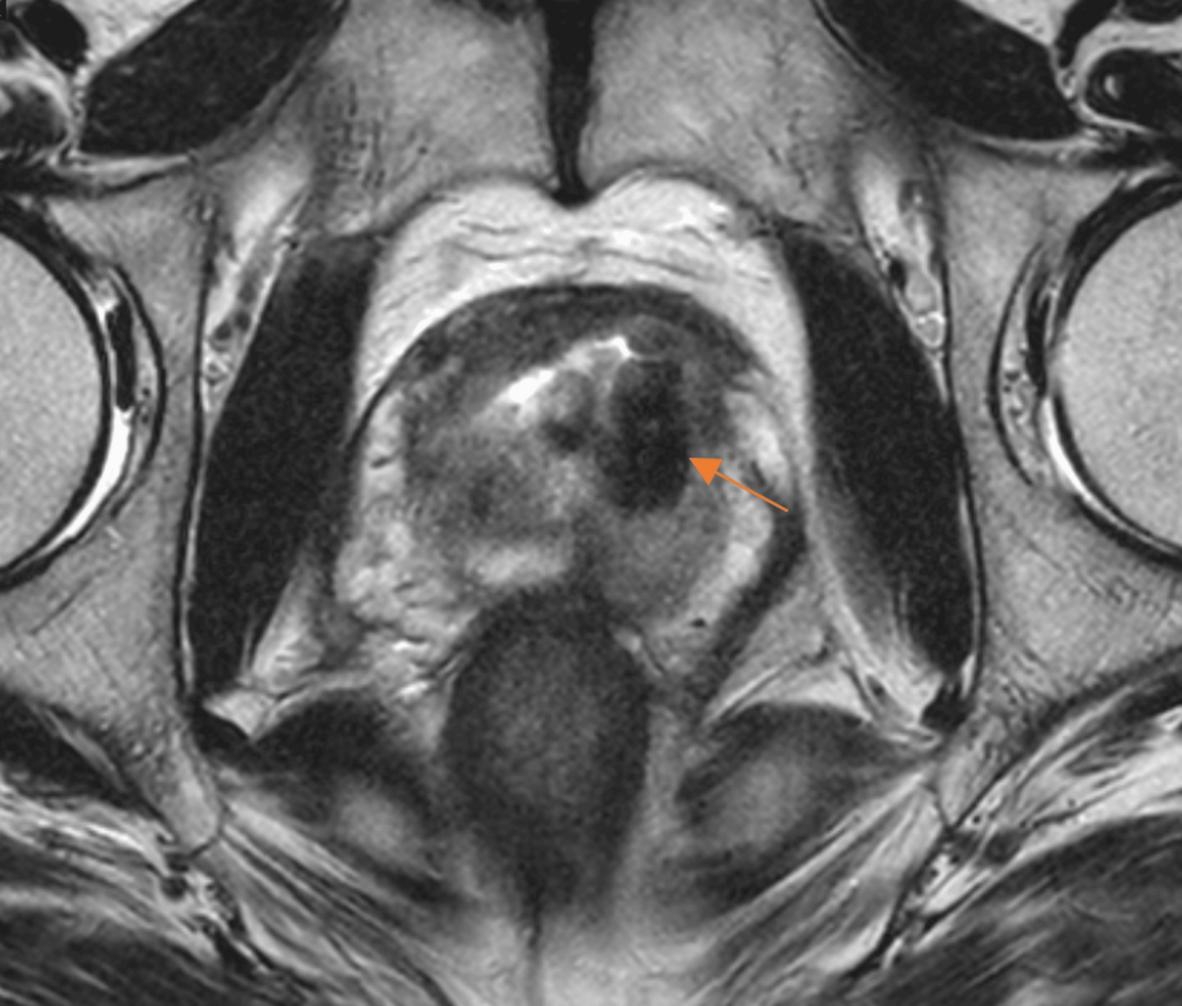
Prostate MRI after HoLEP. T2-weighted multiparametric prostate MRI demonstrating a large defect consistent with sequelae of prior HOLEP. No distinct mass lesion or pathological lymphadenopathy is observed. HoLEP, Holmium laser enucleation of the prostate; MRI, magnetic resonance imaging
